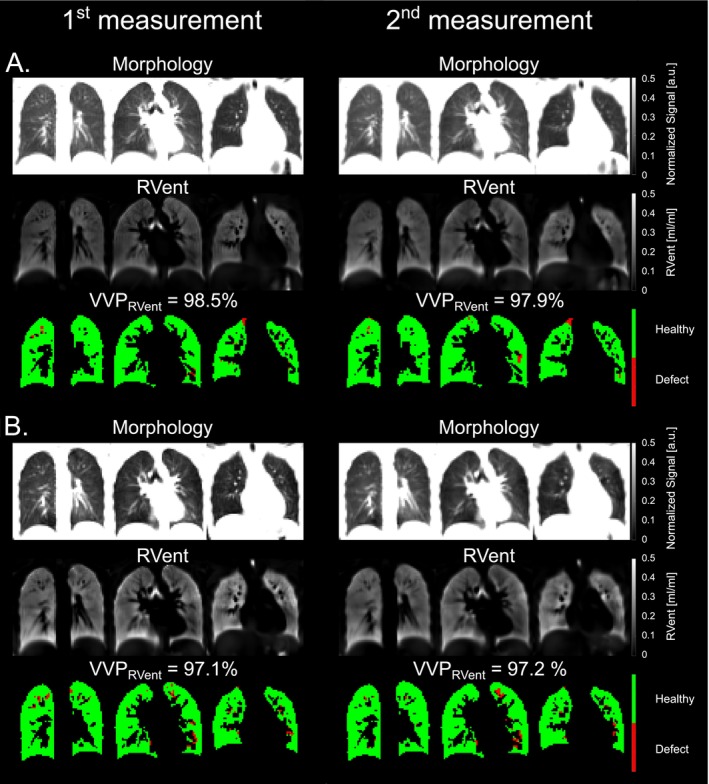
Repeatability comparison of static 3D PREFUL MRI parameters at Center 1 at both field strengths. Representative morphological, static regional ventilation (RVent) maps and corresponding binary ventilated volume maps (A—1.5 T Center 1, B—3 T Center 1) for a healthy volunteer (26‐year‐old female, FEV_1_ = 99% pred.) of the first (left) and second (right) 3D PREFUL measurements. Mean RVent of the first measurement for the whole lung volume was 0.15 mL/mL (coefficient of variation [CoV] of 28%) at 1.5 T and 0.22 mL/mL (CoV of 31%) at 3 T. Mean RVent of the second measurement for the whole lung volume was 0.17 mL/mL (CoV of 31%) at 1.5 T and 0.20 mL/mL (CoV of 30%) at 3 T. VVP_RVent_, ventilated volume percentage based on RVent parameter.
